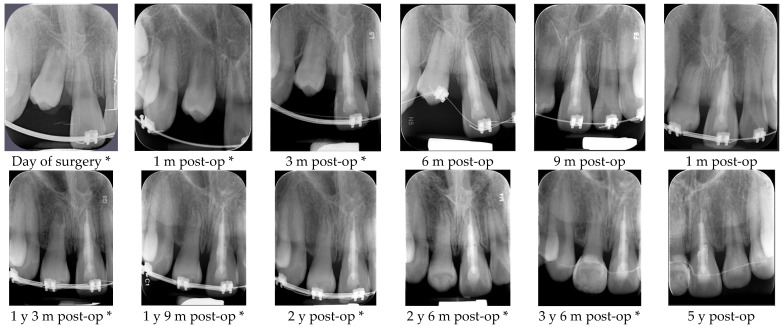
Sequential periapical radiographs of tooth 21 in the five years following the autotransplantation. Due to a lack of clearer radiographs, some are copies of the entries in Figure 17, marked with *. Time elapsed in years (y) and months (m). Dimensions: 3.05 × 4 cm.
